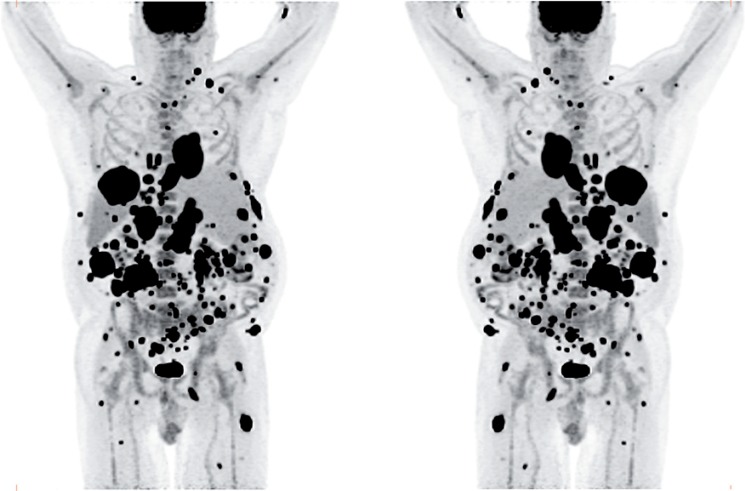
Maximum intensity projection (MIP) images in anterior and posterior views of the ^18^F-FDG PET/CT images showing multiple hypermetabolic foci of increased FDG uptake throughout the body, most likely representing widespread metastatic disease.
